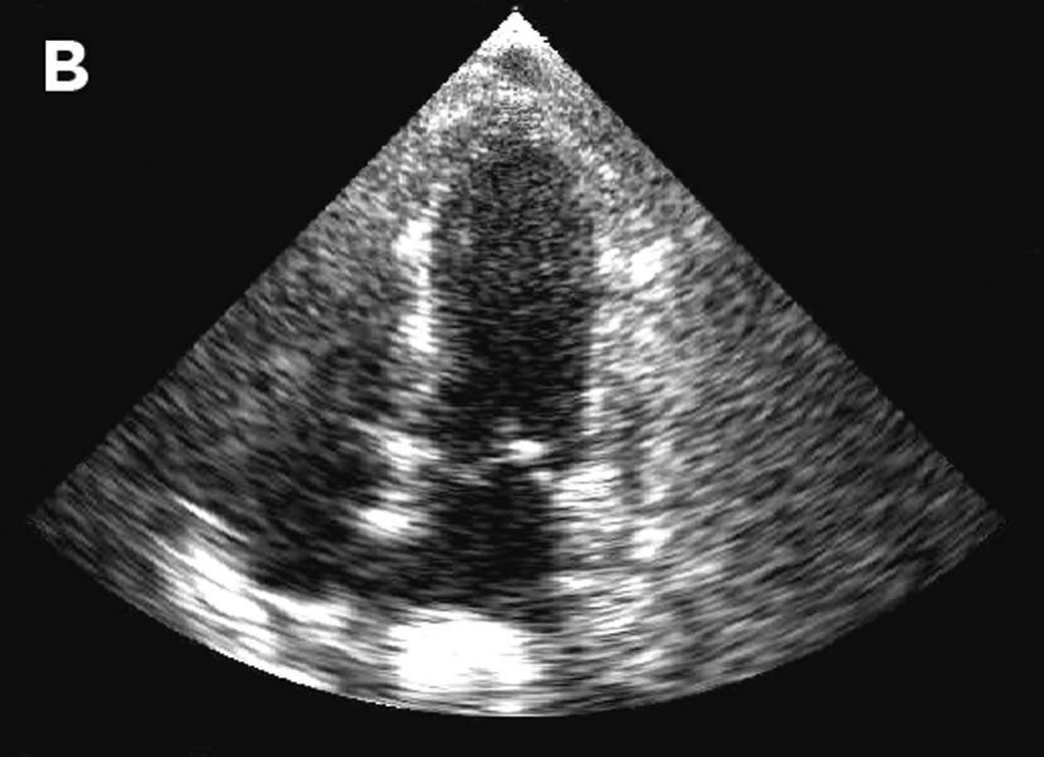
Resolution of left ventricular function on repeat echocardiograph 6 days later.
